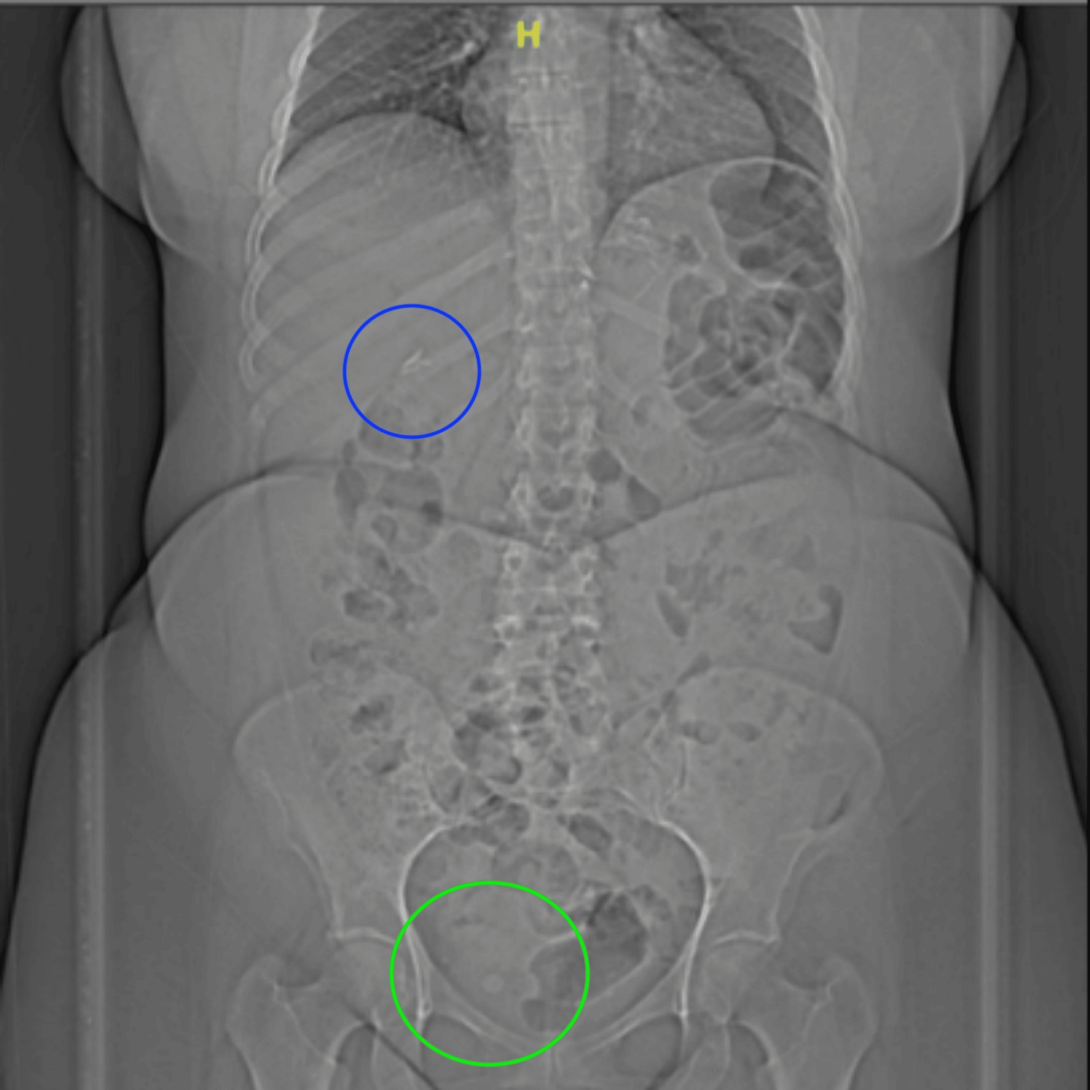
KUB X-ray revealed multiple surgical clips in the mid-abdomen and right upper quadrant (blue circle). Rounded radiopaque structures were noted in the pelvis, likely representing phleboliths (green circle). KUB, kidney, ureter, and bladder
